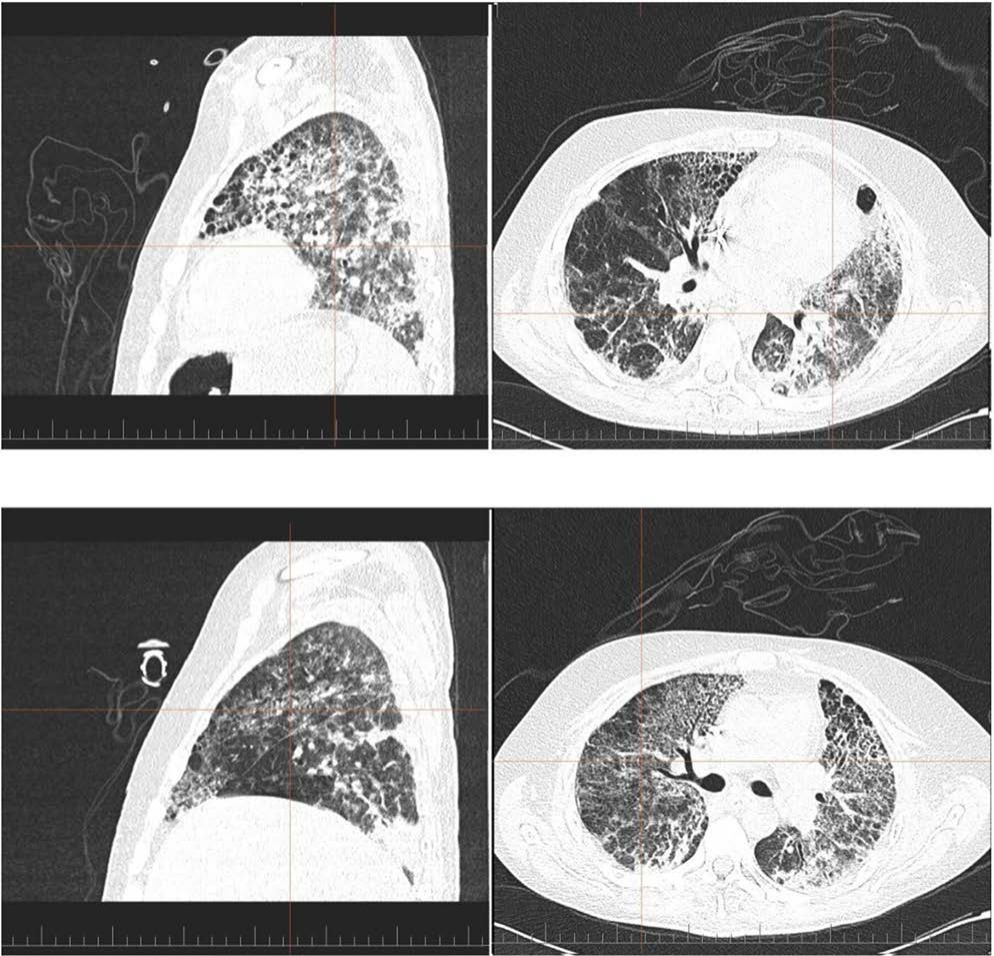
CT sagittal and a coronal view of lungs. In the upper row, the red cross marker is focused on the left lung. In lower row, the red cross marker is focused on the right lung. CT = computed tomography.
